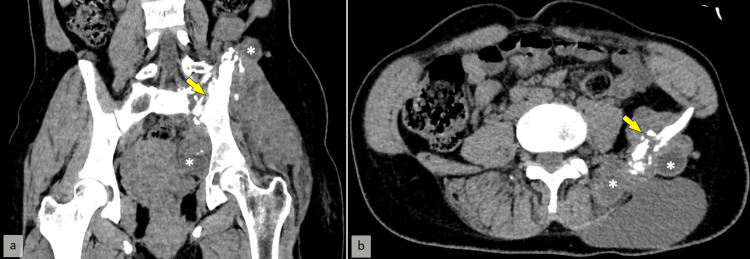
Coronal (a) and axial (b) CT scans show an enlarged left psoas muscle with a hypodense cystic lesion (*) and clear destruction of the left iliac wing (yellow arrow).
